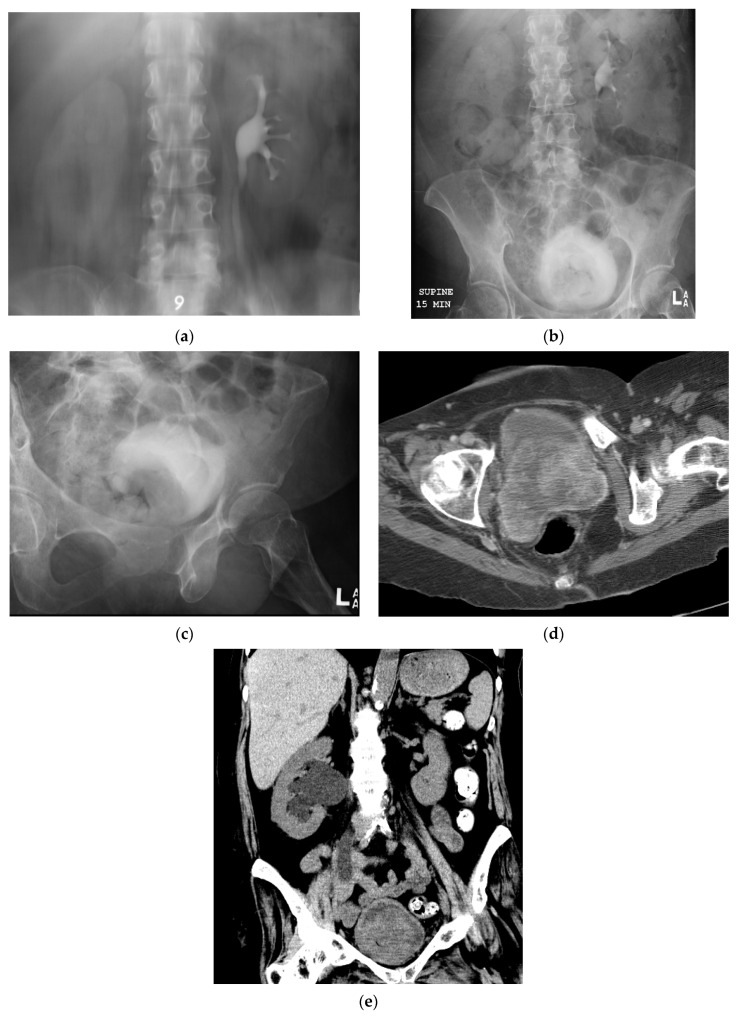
IVP and CT images of locally advanced bladder cancer. (a) IVP image of the upper urinary tract at 9-min is without filling defect in the left collecting system to suggest left-sided upper tract disease. The right collecting system is poorly opacified secondary to hydroureteronephrosis, better depicted on CT. (b) IVP image at 15 min shows a large filling defect in the bladder. (c) Magnified oblique view of the bladder better show a posterior filling defect. (d) Axial portal venous phase CT image of the pelvis shows the corresponding mass in the posterior bladder which extends posteriorly to involve the vagina. (e) Coronal portal venous phase CT image shows the bladder mass causing right hydrouteronephrosis.
